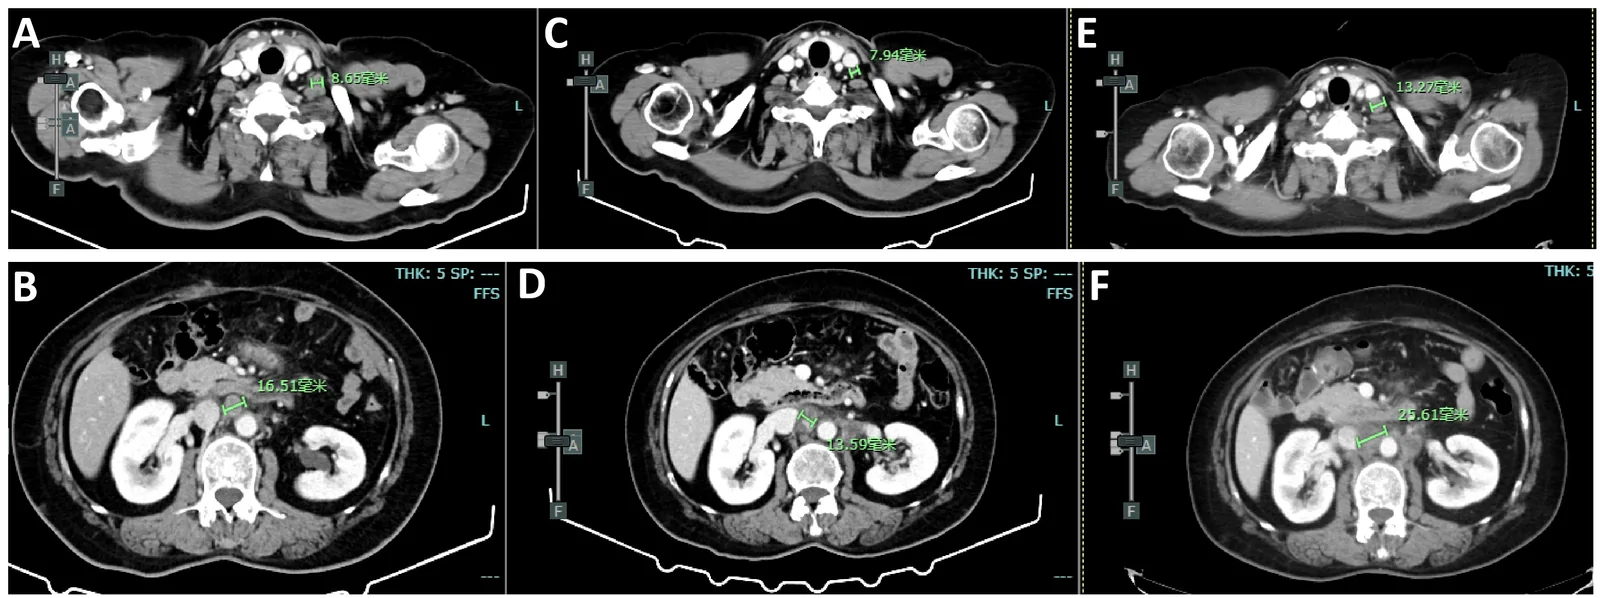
Serial chest CT images during third-line camrelizumab plus surufatinib therapy. (A, D) Baseline (July 1, 2023). Target lesion: 1.6 cm. (B, E) Best response (October 2, 2023). Target lesion reduced to 1.3 cm (−18.8%); per RECIST, best overall response: stable disease (SD). (C, F) Progression (December 18, 2023). Target lesion enlarged to 2.6 cm (+62.5%) with new/progressive non-target lesions; per RECIST, progressive disease (PD). Scan dates are shown in each panel. All images are at comparable anatomical levels.
